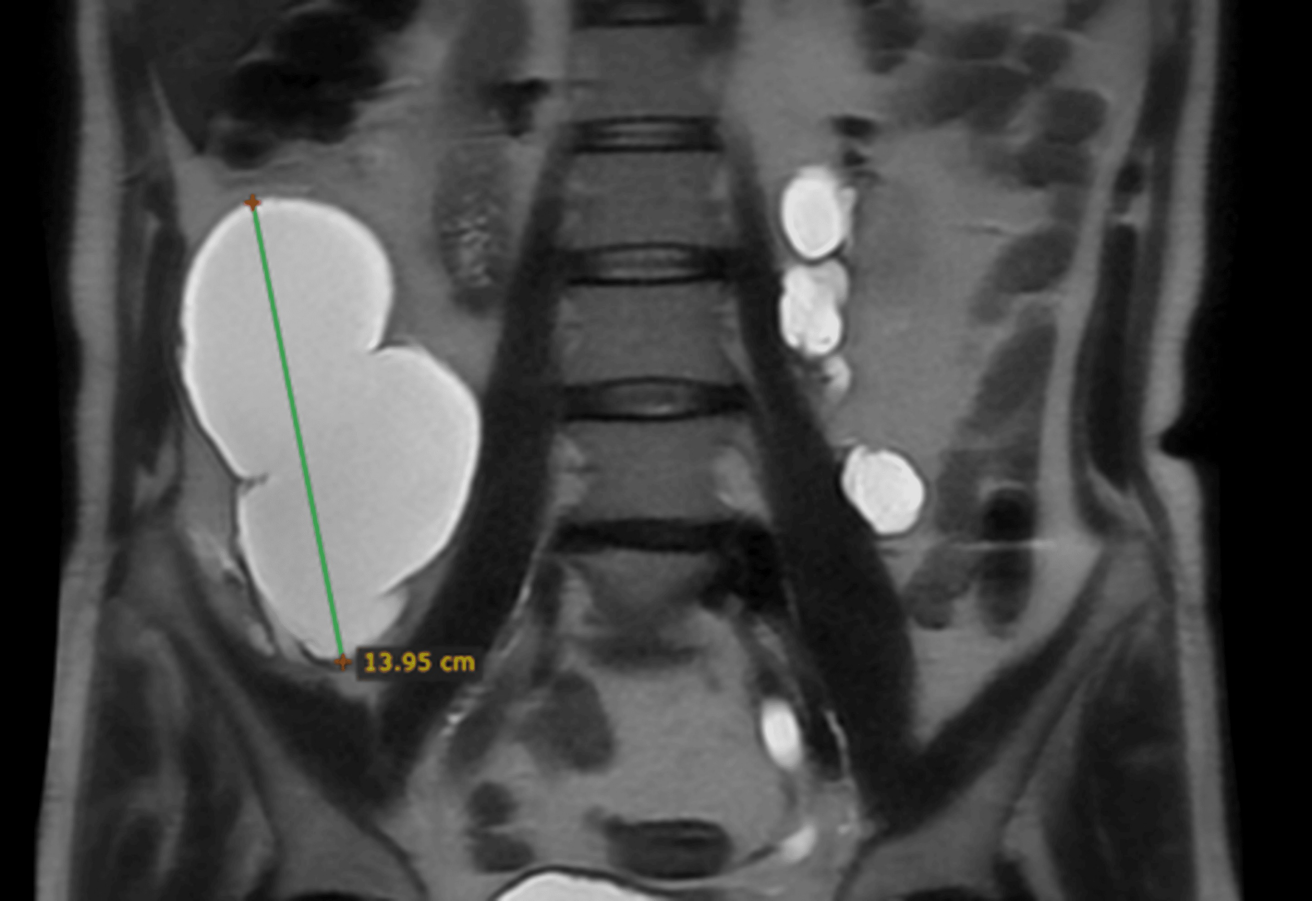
Non-contrast T2-weighted MRI of the abdomen (coronal view) showing a hyperintense collection in the right lumbar region measuring 13.9 cm in the maximum craniocaudal dimension (blue arrow).
